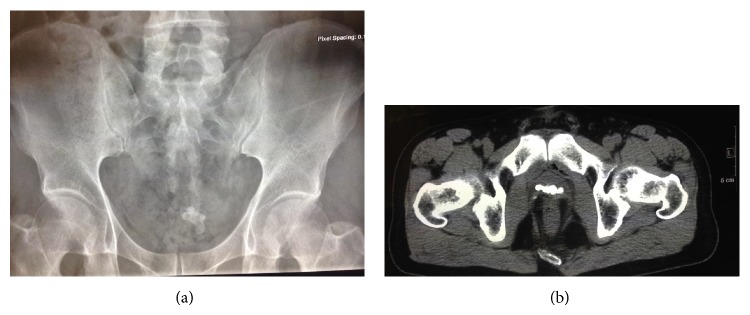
Pelvic X-ray (a) and computed tomography (b) demonstrate an approximately 2.8 cm calculus in the neobladder.
